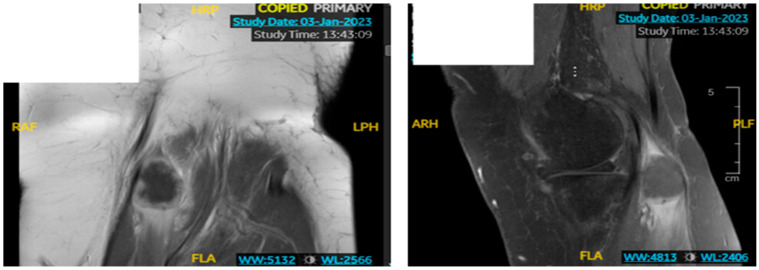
Left lower extremity MRI (Magnetic Resonance Imaging) showed a 1.8 × 1.9 × 2.6 cm circumscribed mass in the medial head of the gastrocnemius muscle at the level of the tibial plateau.
